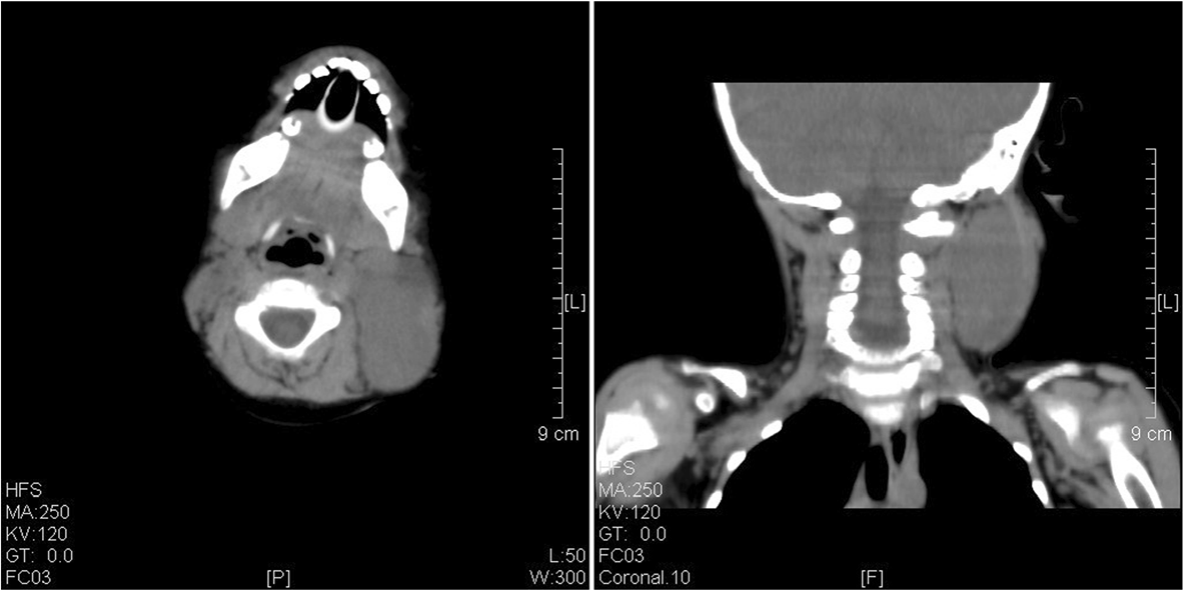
A CT scan showed a haematoma under the sternocleidomastoid muscle in case 2.
